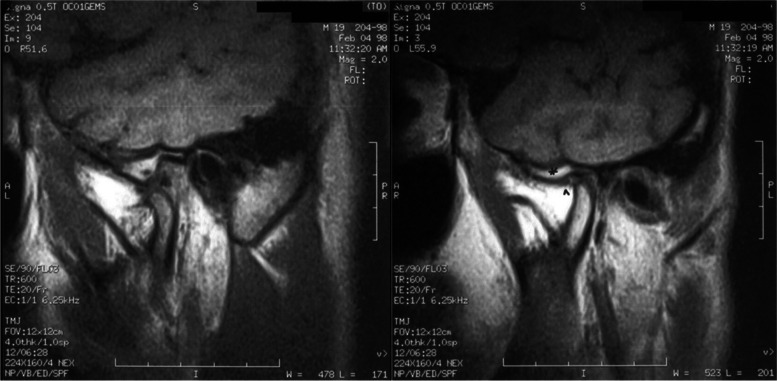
Magnetic resonance imaging (MRI) sagittal T1 weighted scans. a right temporomandibular joint (TMJ), open mouth: physiologic joint with normal condylar excursion. b left TMJ, open mouth: notice a less pronounced anterior tubercle of the temporal bone and a shallow articular cavity (*); the articular disk is hypoplastic (^); complete condyle immobility is evident
